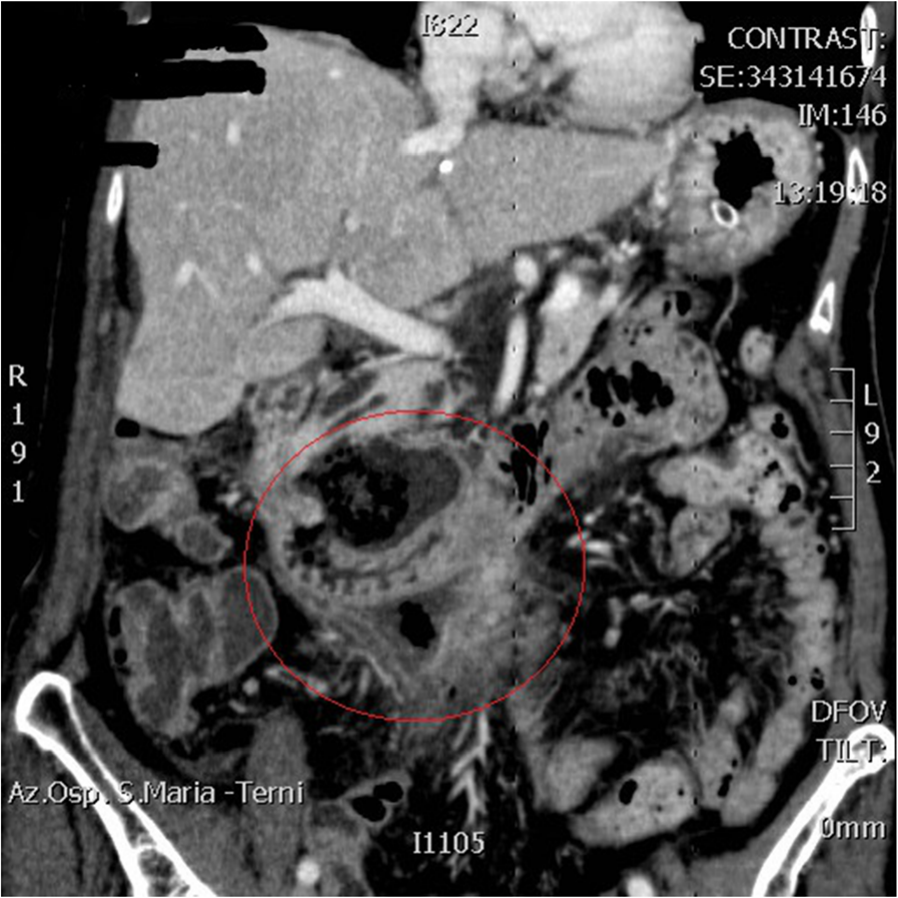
CT after three days from the admission.
